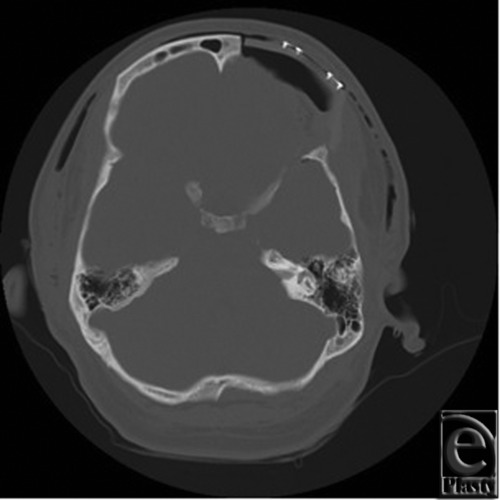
Postoperative computed tomographic scan showing polyetheretherketone implant in place at the frontal bone.
